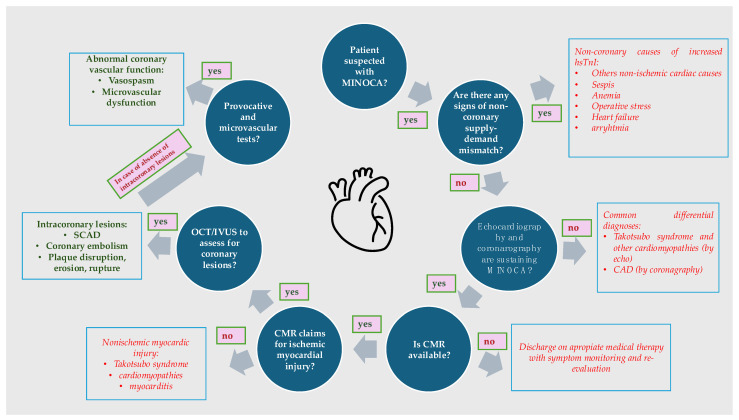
Diagnostic tools in patients suspected of MINOCA. CAD: coronary artery disease; CMR: cardiac magnetic resonance; IVUS: intravascular ultrasound; OCT: optical coherence tomography; SCAD: spontaneous coronary artery dissection.
